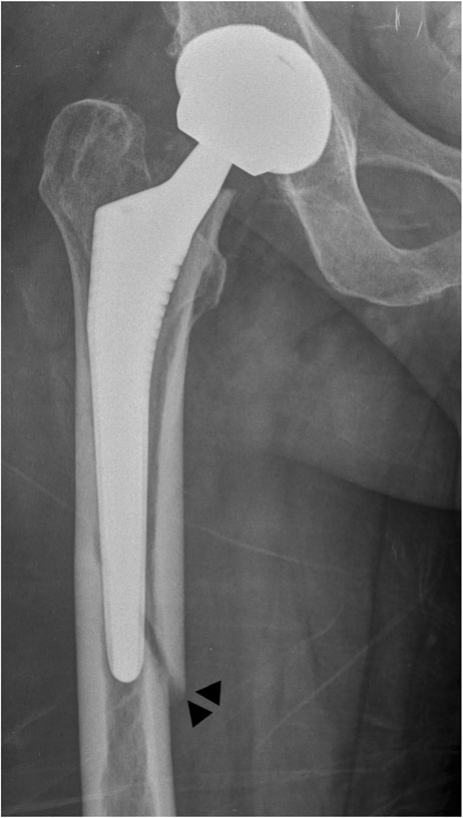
AP radiograph. Cementless total hip arthroplasty. Periprosthetic fracture in Gruen zone 5 (*arrowheads*), Vancouver type B1 fracture
